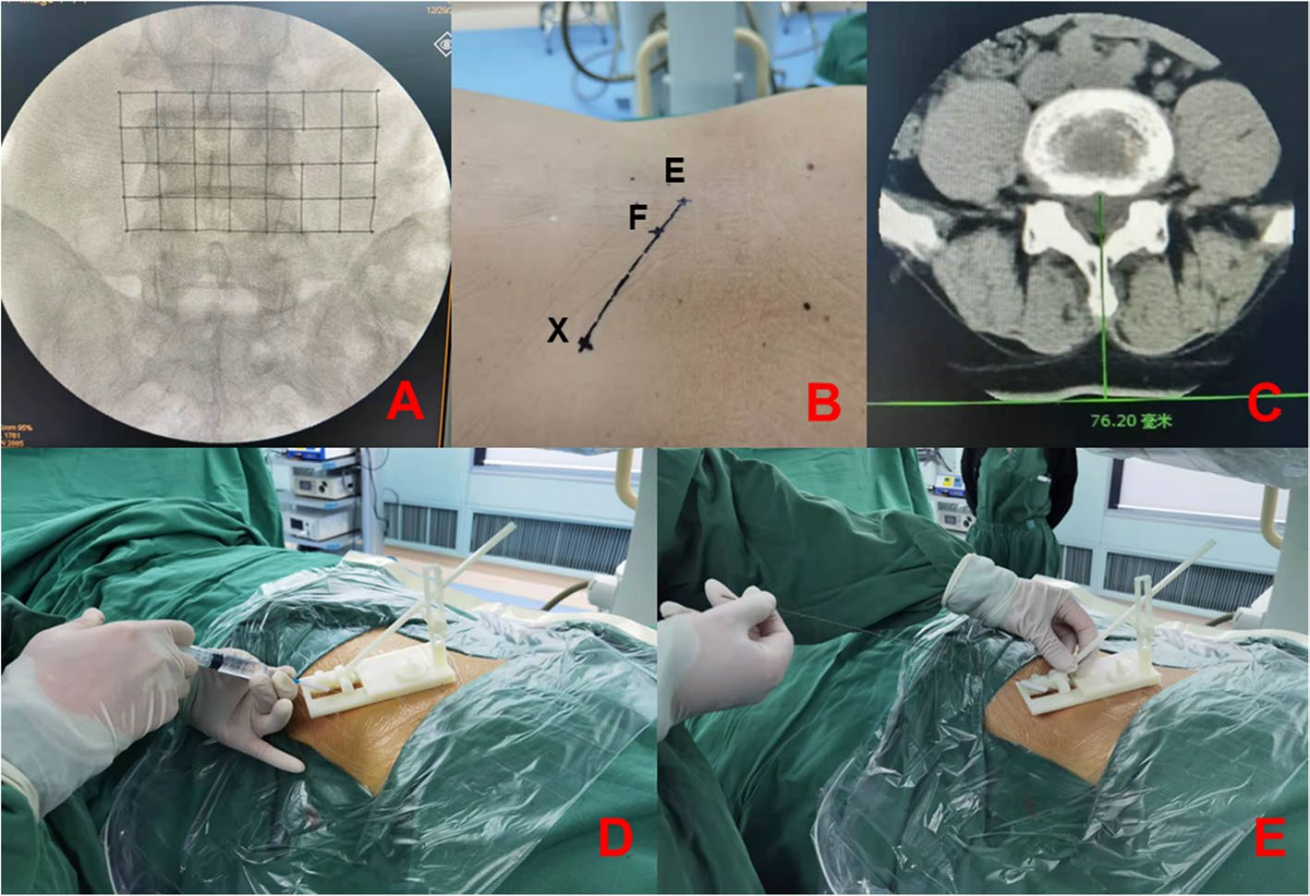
Application of the body surface-assisting puncture device during surgery. A, B The iron positioning grid was used to locate the puncture point. C To measure the vertical distance between the midpoint of the posterior upper margin of the lower vertebral body to skin on the CT cross-section. D Adjust the height of the body surface-assisting puncture device according to the measured distance to determine the puncture path, then the anesthesia was infiltrated layer by layer. E Use the needle to puncture to the target point through the body surface-assisting puncture device
